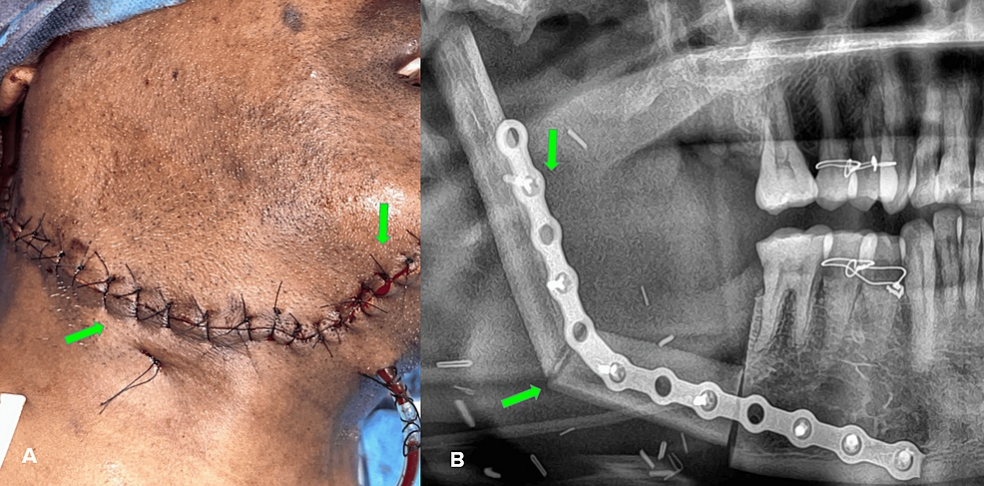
Follow-up images: clinical (A) and orthopantomogram (B) A - Clinical picture showing the surgical wound closed with sutures B - Orthopantomogram showing the fibula graft along with the reconstruction plate
